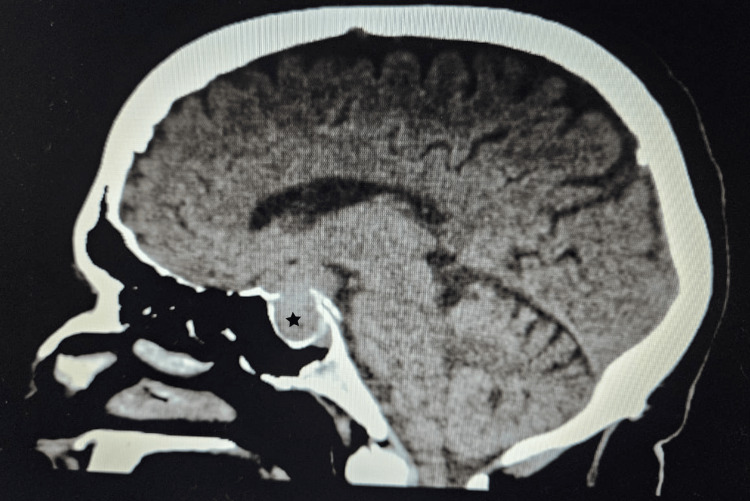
Magnetic resonance imaging (MRI) of the brain depicting a 1.3x1.3x3.2 cm pituitary macroadenoma (star)
